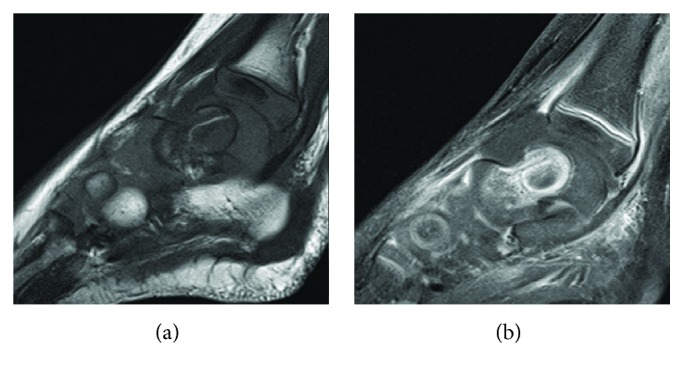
MRI T1-weighted lateral (a) and T1 postcontrast lateral (b) cuts of the ankle.
